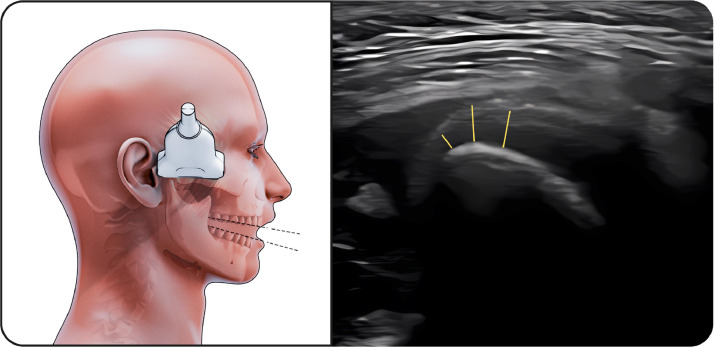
Anterior capsule-condyle distances. During ultrasonographic examination using a transverse scan, mouth opening results in anterior translation of the hyperechoic mandibular condyle, accompanied by widening of the anterior capsule-condyle distance. The cortical surface of the condyle appears as a hyperechoic line with posterior acoustic shadowing, while the joint capsule is visualized as a hyperechoic structure anterior to the condyle. The articular cartilage is identified as a thin, hypoechoic layer covering the condylar surface and is visible between the hyperechoic cortical bone and the joint space, the relative position of which changes during mouth opening as the mandibular condyle translates anteriorly. These structures allow standardized assessment of anterior joint space width and dynamic changes during mandibular movement.
